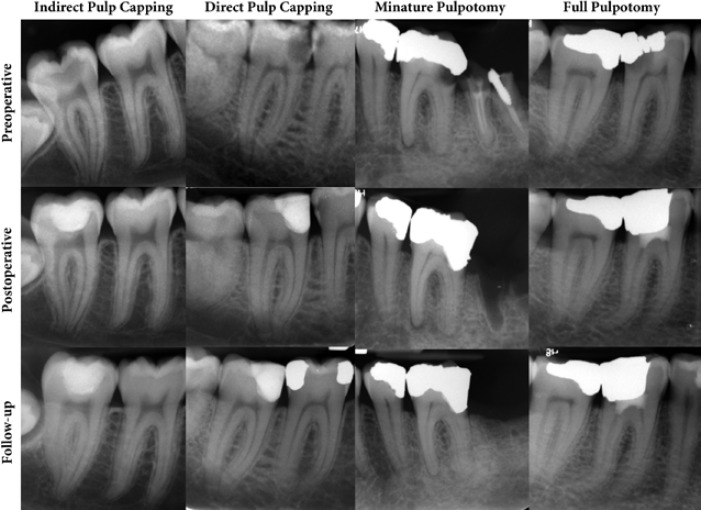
Preoperative, postoperative and follow-up radiographies of four mature molars treated with indirect pulp capping, direct pulp capping, miniature pulpotomy and coronal pulpotomy. In all cases, the widening of the periodontal ligament and periapical lucency is visible in the preoperative radiographs, which totally vanished in the follow-up clichés. Note that all cases were diagnosed with clinical signs of irreversible pulpitis
